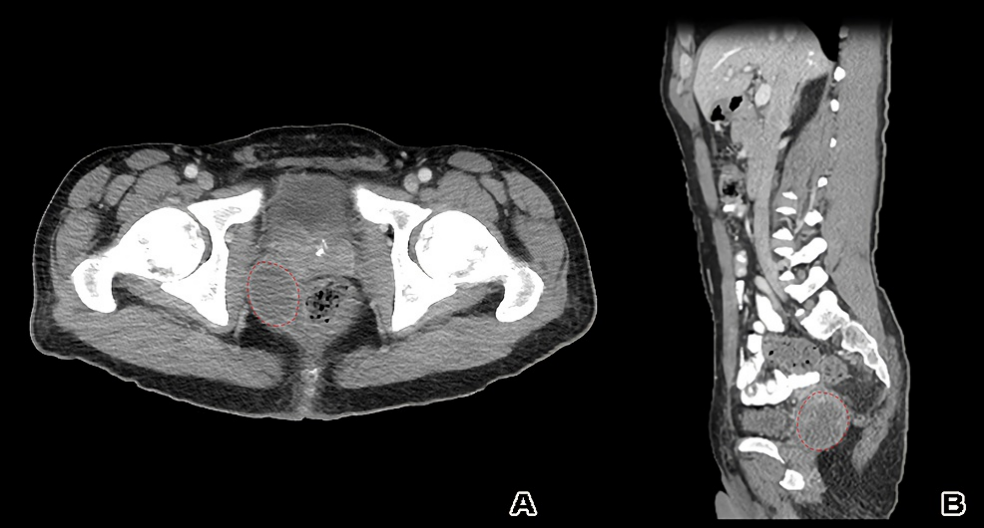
(A) Axial and (B) sagittal CT scan, at the follow-up interval after three cycles of chemotherapy, showed reduction in tumor size (6.5 x 5 x 5 cm) and mass effect but still rectum and prostate gland indentation. The dashed red lines mark the tumor.
